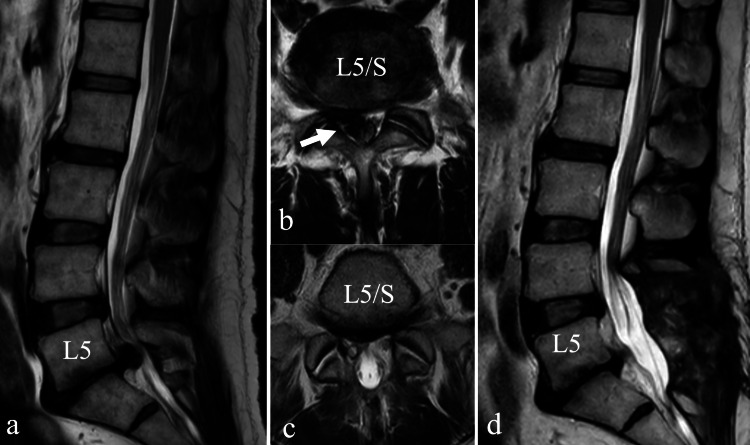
Preoperative (a, b) and postoperative (c, d) MRI of case 2 a. Preoperative MRI T2-weighted sagittal section showed stenosis at L4/5 and a large herniation protruding at L5/S. b. Preoperative MRI T2-weighted transverse image showed a shift of the dural tube to the left due to the herniation (arrow) that prolapsed to the right at L5/S. c. Postoperative MRI T2-weighted transverse section showed the disappearance of the herniation, widening of the dural tube, and the decompressed right S1 nerve root. d. Postoperative MRI T2-weighted sagittal section showed that the stenosis of L4/5 and 5/S had been decompressed, and the hernia had disappeared.
